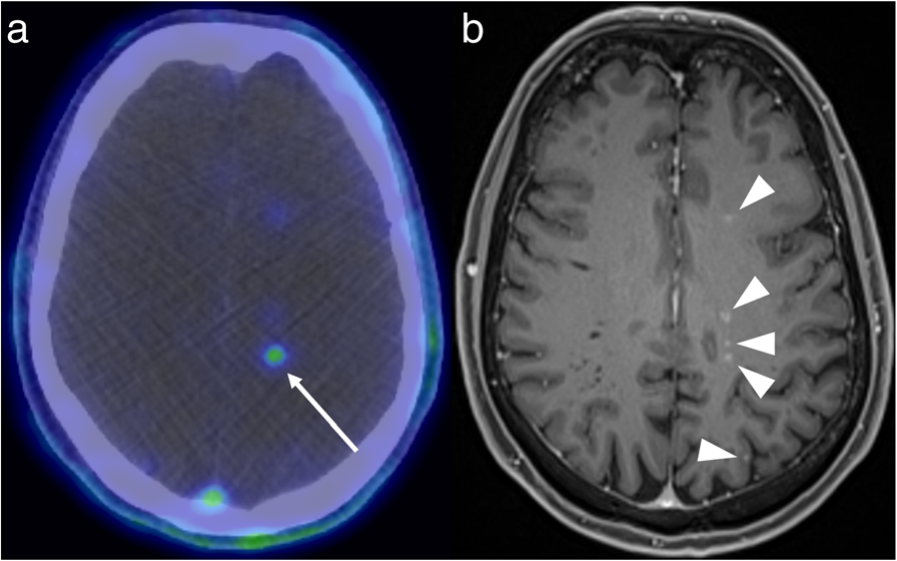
PSMA-PET (a) in a patient with prostate cancer shows a focus of high uptake intracranially (arrow). The post-contrast MRI (b), however, demonstrates much more widespread intracranial metastatic disease (arrowheads)
